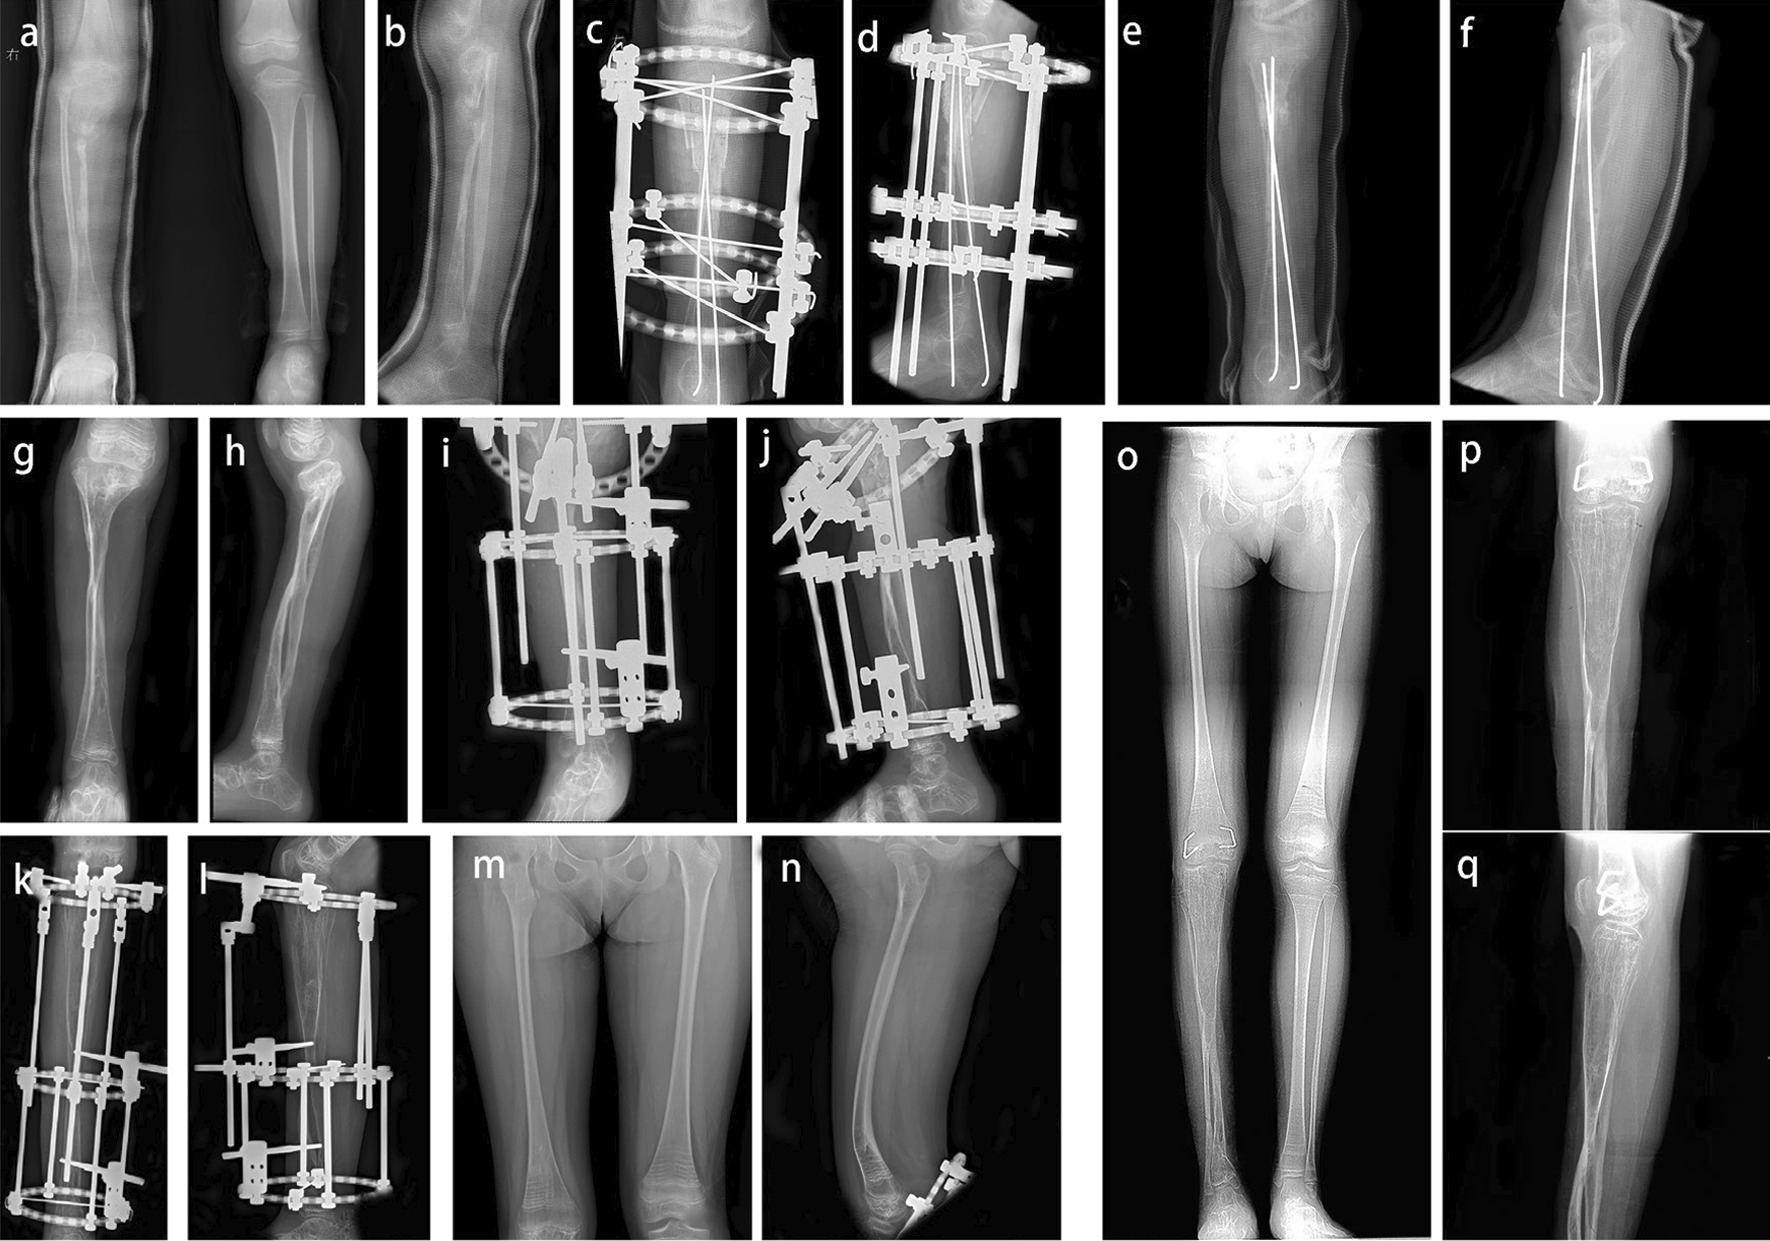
Case 10: Radiographic presentation of a 5-year-old girl with right congenital pseudoarthrosis of the tibia treated via debridement, autogenous bone grafting, and external fixation prior to ipsilateral fibula transfer. a, b Preoperative anteroposterior and lateral radiographs: The tibial lesion was approximately 14.7 cm, and the remaining normal tibia was shorter than the contralateral side by approximately 18.5 cm. c, d Postoperative radiograph with ipsilateral fibular transfer. e, f 5 months after the ipsilateral fibular transfer. g, h 3.7 years postoperative follow-up, a deformity of the posterior arch of the proximal tibia can also be seen. i, j Due to the proximal posterior arch of the tibia, she underwent an oblique osteotomy and a gradual lengthening of the fibula via external fixation. k, l Radiographs were again taken 1.3 year after the proximal epiphysis osteotomy and fixation. m, n 7 months after the fracture of the right distal femur, the distal femur showed an anterior arch deformity. o–q 6 years postoperative follow-up, significant hypertrophy of the transplanted fibula can be seen
